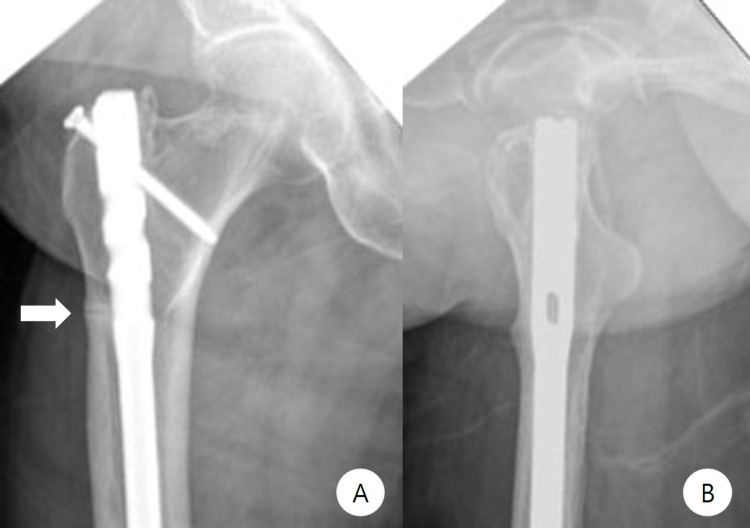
Preoperative imaging - case 2 Preoperative anteroposterior (A) and lateral (B) radiographs show incomplete fracture line (white arrow) and anterior cortical thickening at the lower border of the lesser trochanter. The images show complete healing of the previous fracture
